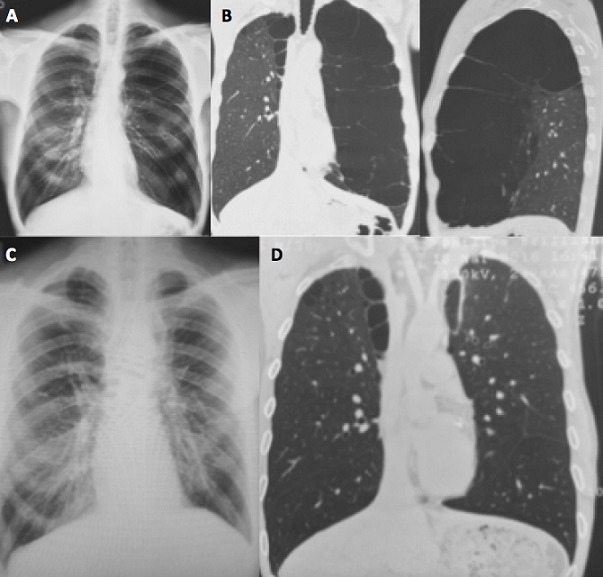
A) preoperative chest X-Ray. Left pulmonary hyperlucency; B) preoperative chest CT. Giant emphysematous bulla with thin-walled partitions inside replacing almost the entire upper lobe; C) postoperative chest X-Ray. Complete pulmonary expansion; D) postoperative chest CT
